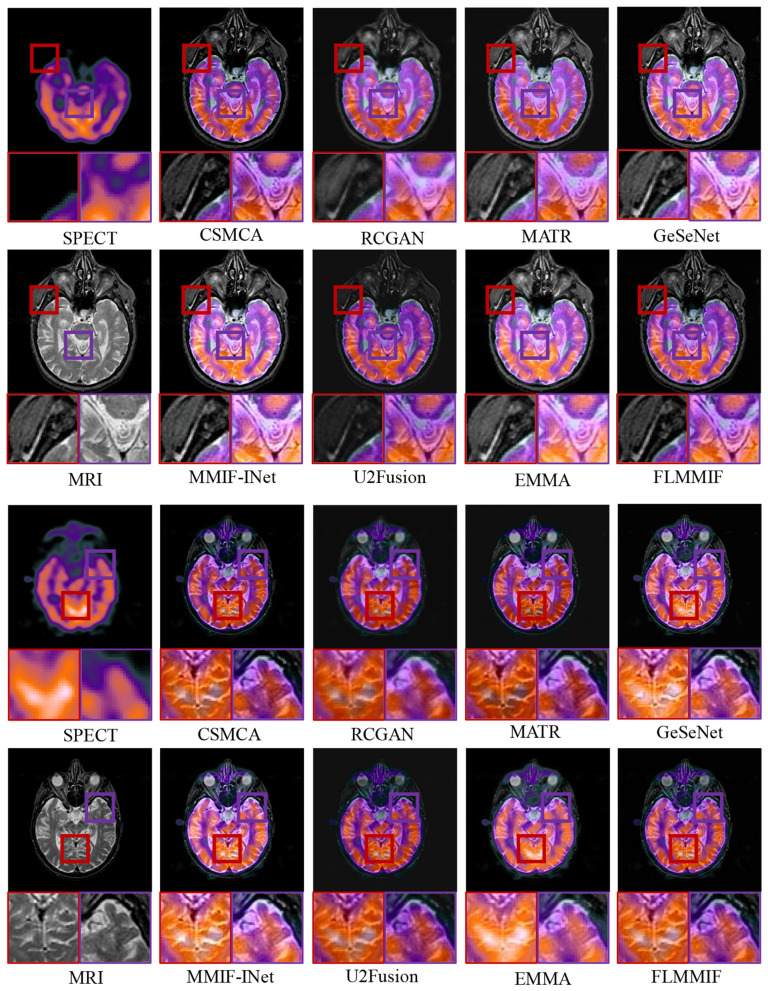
Qualitative assessment on SPECT-MRI dataset.
